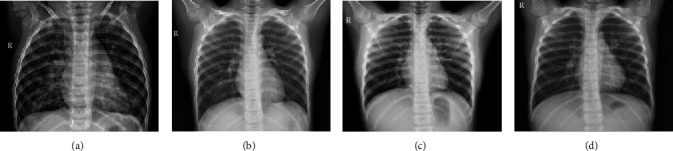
(a and c) The medical images of the pneumonia chest X-ray in two hospitals, respectively, and (b and d) the medical images of the chest X-ray with confirmed pneumonia.
